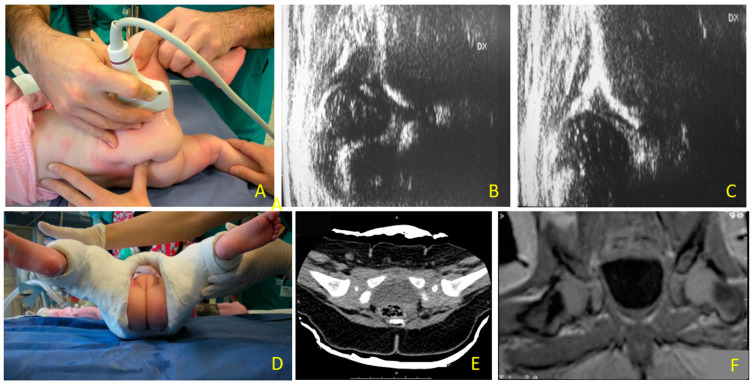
Hip dislocation: (A) ultrasound evaluation with lateral approach to the hip joint according to Graf technique during reduction maneuver; (B) type IV hip; (C) femoral head in the acetabular fossa after closed reduction, before performing a pelvi-podalic spica cast (SC); (D) the SC has been applied. With SC, Graf’s technique cannot be applied anymore, to check if the hip is reduced or not, (E) to verify the position of the femoral head in SC, computer tomography (CT) or (F) magnetic resonance (MR) can be used.
